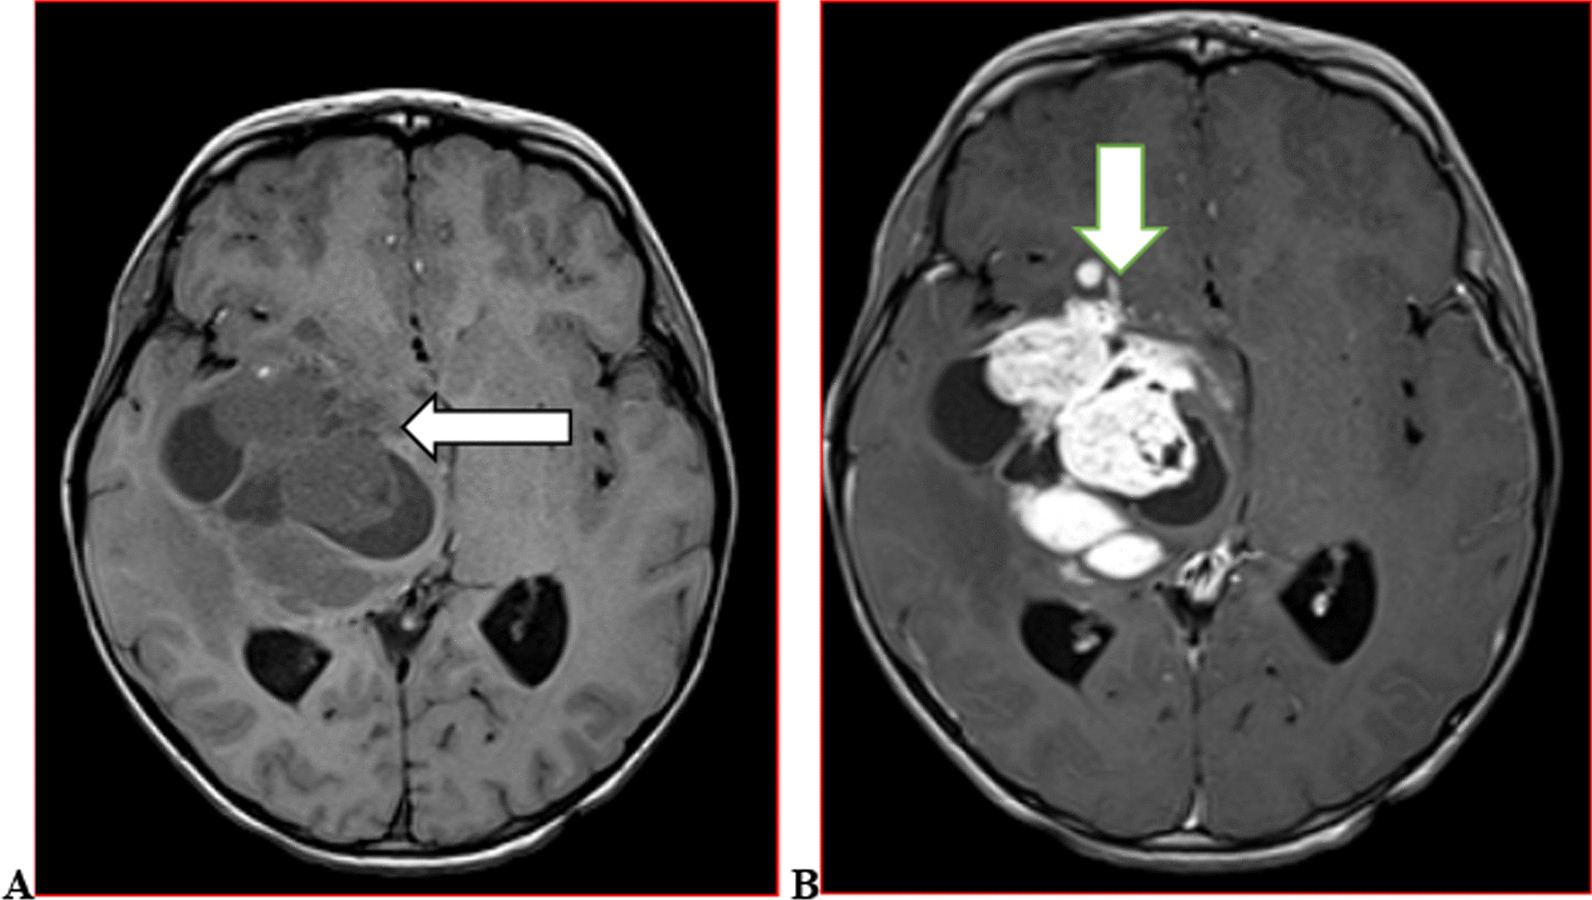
Six year old male with epilepsy. Axial T1 weighted brain MR images pre (A) and post (B) gadolinium contrast demonstrating a heterogenous mass in the right temporal lobe with extension to ipsilateral basal ganglia and thalamus (arrows) with avid enhancement of the solid component in a case of a tumor
